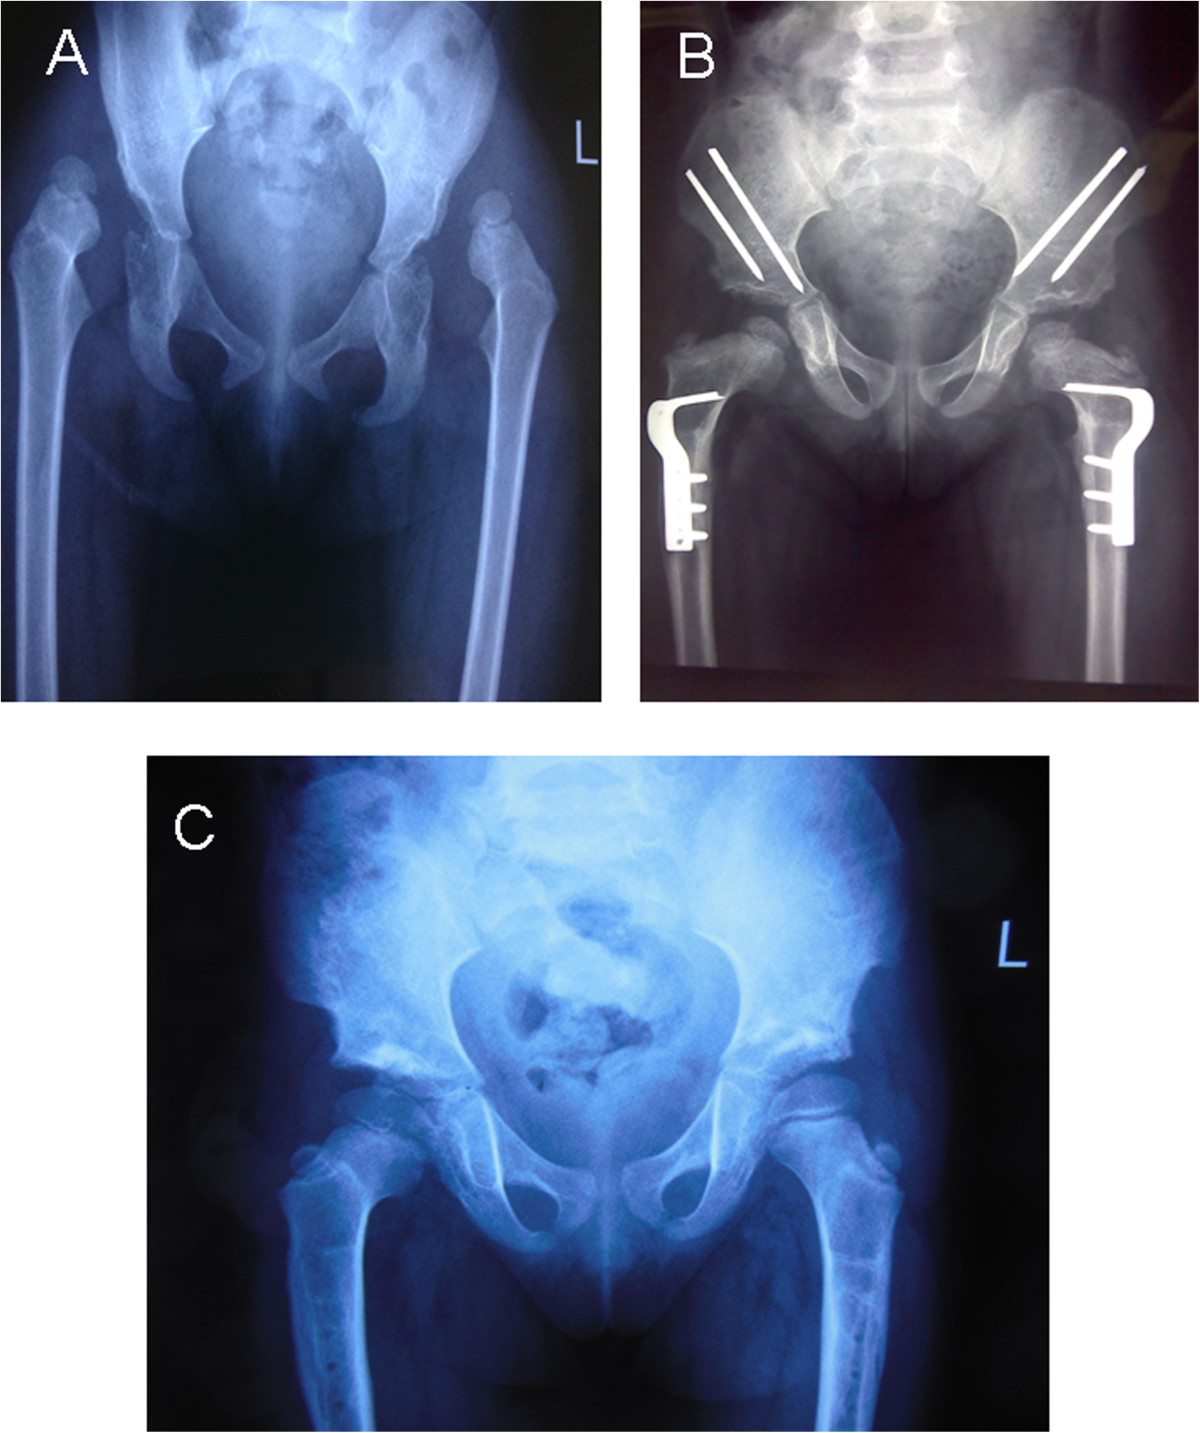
Shows a typical X-ray in a bilateral DDH, both before operation and at the follow up examination. A. Female patient aged 3.8 years with bilateral DDH. Plain X-ray anteroposterior (AP) view. B. Plain X-ray AP view 1 year after one-stage operation, showing good containment of the femoral head. C. AP view 3.9 years postoperatively with excellent clinical and radiographic results.
